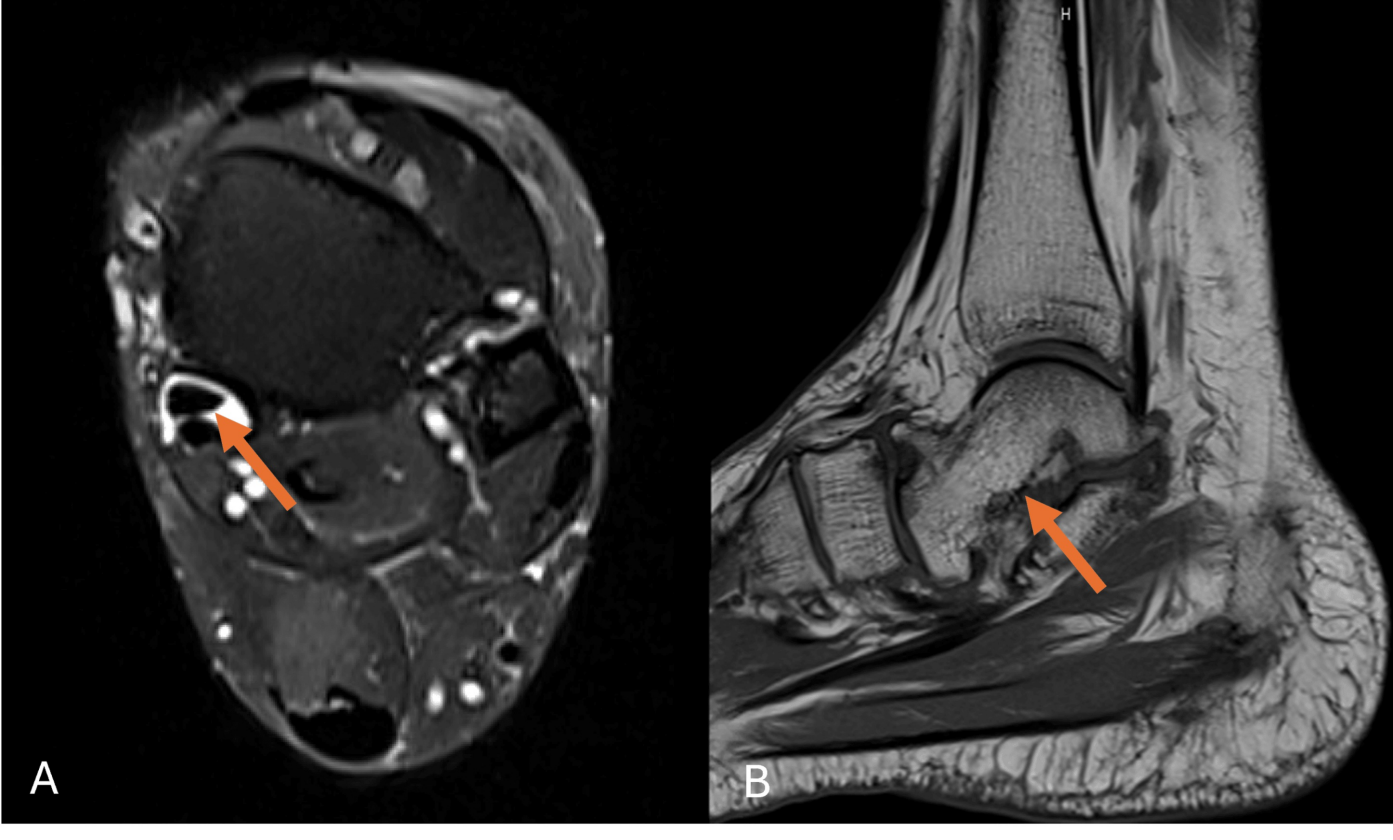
(A) T2 fat-saturated axial-weighted MRI and (B) T1 sagittal-weighted MRI (A) demonstrating posterior tibial tenosynovitis and (B) showing talar deformity and subtalar degeneration (arrows) MRI: magnetic resonance imaging
